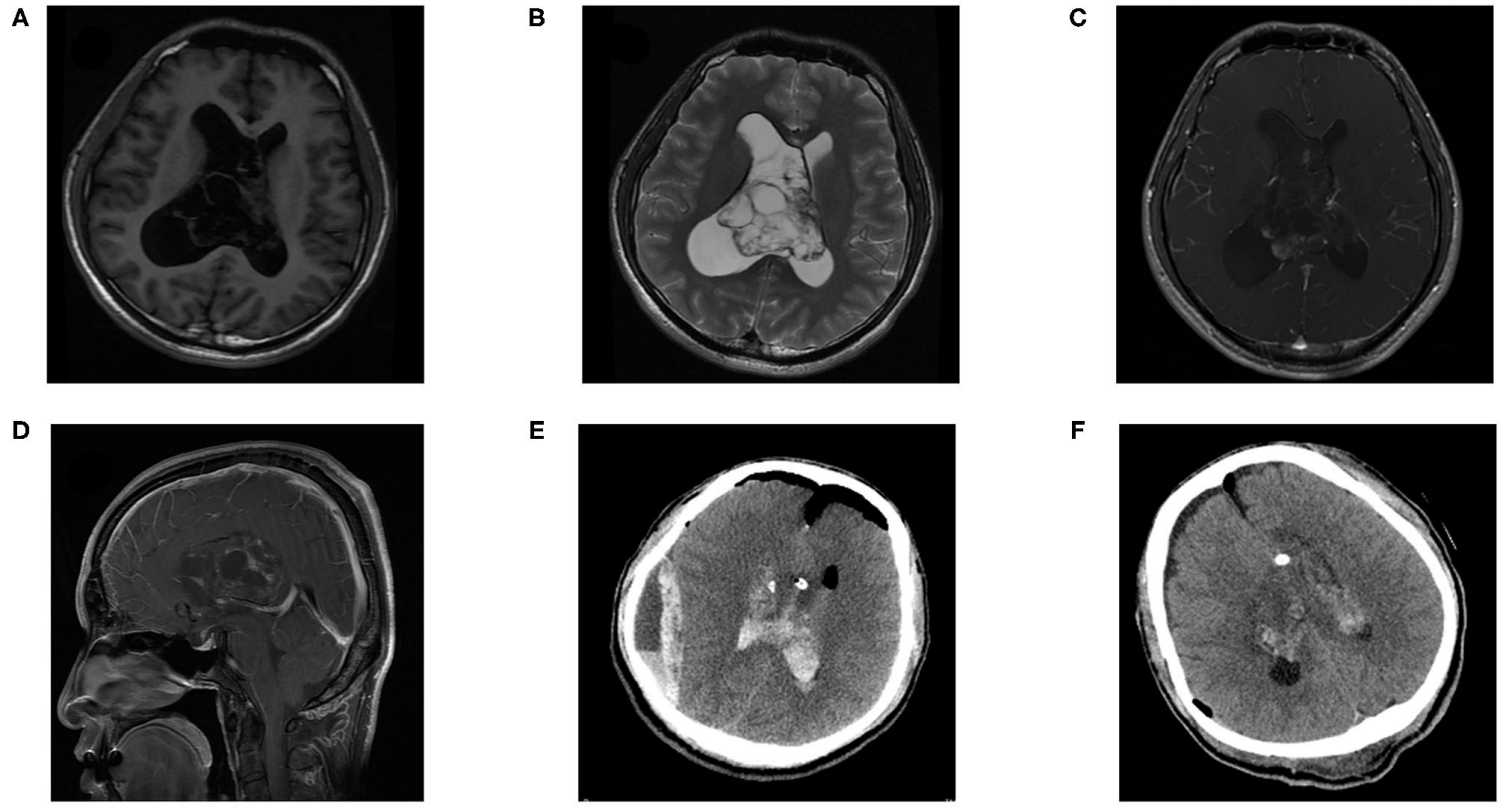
A 27-year-old female patient suffered epidural hematoma with central one day after surgery. Pre-operative (A) T1 weighted axial sequence, (B) T2-weighted axial sequence, (C) T1-enhanced axial sequence, (D) T1-enhanced sagittal sequence, MRI scans showing a 61 × 65 × 37 mm-sized mass in bilateral lateral ventricles, (E) CT revealed an epidural and cavity hematoma after the tumor resection. (F) CT showed that the hematoma had been cleared, indicated that there was residual tumor.
